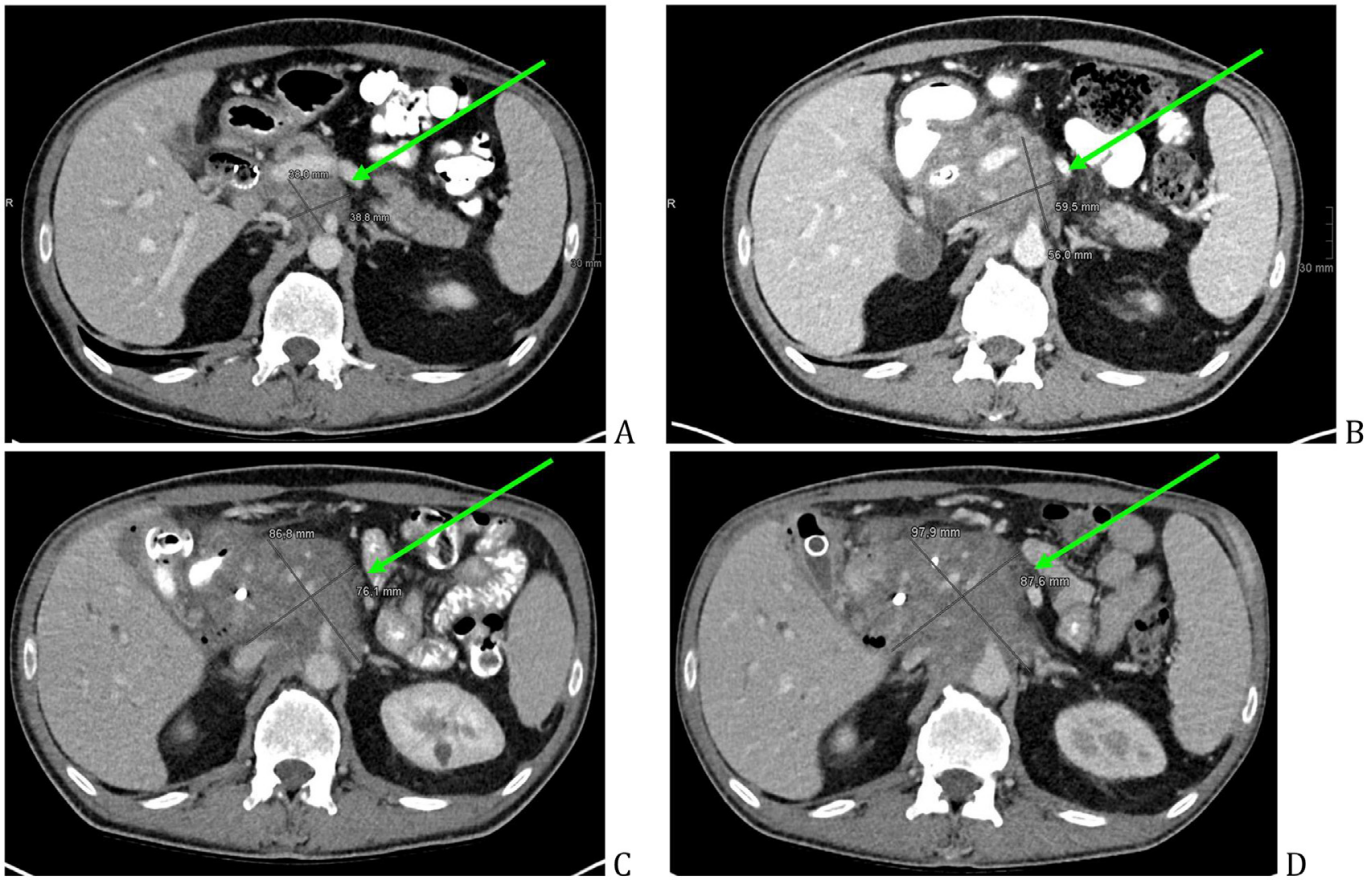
Follow-up CT within 6 months, pancreatic head mass (green arrow) size increase. Initial work-up - 38 mm (A), follow-up after 3 months—59 mm (B), follow-up after 5 months—86 mm (C), follow-up after 6 months—98 mm (D).
